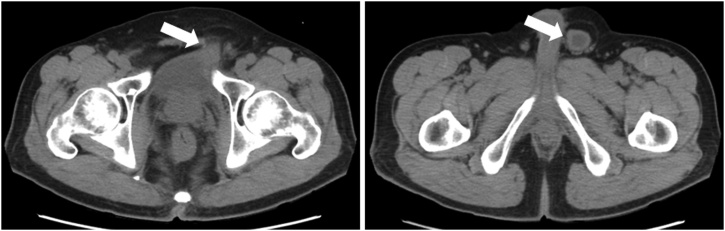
Computed tomography (CT). CT revealed a left inguinal hernia containing a portion of the urinary bladder (arrow).
